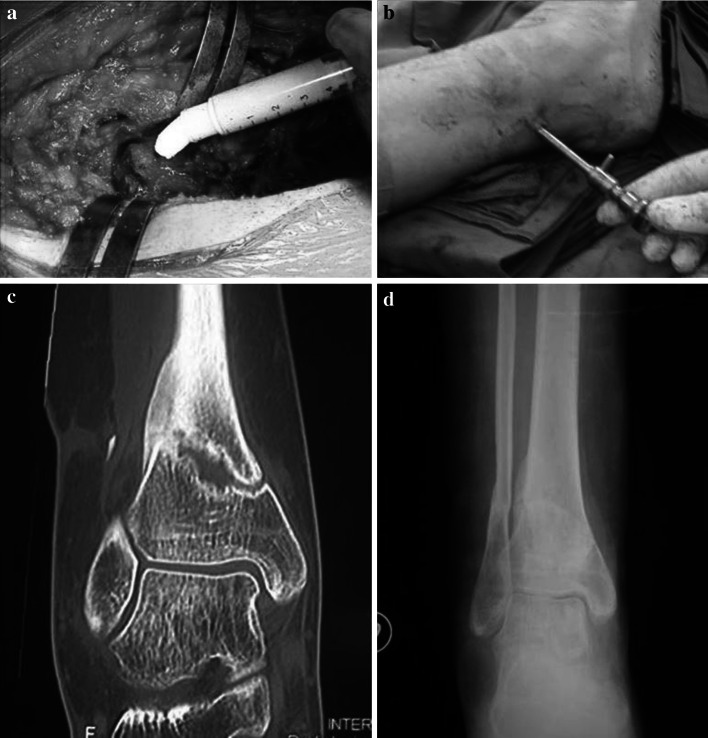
Injectable TCP cement bone substitutes: a–b injectable cements have the advantage to be mouldable and contourable to the bone loss in mininvasive or open surgery; c bone loss in a distal tibial open fracture delayed union (CT scan); d 1 year X-ray control, showed bone consolidation and osseointegration of the TCP cement
